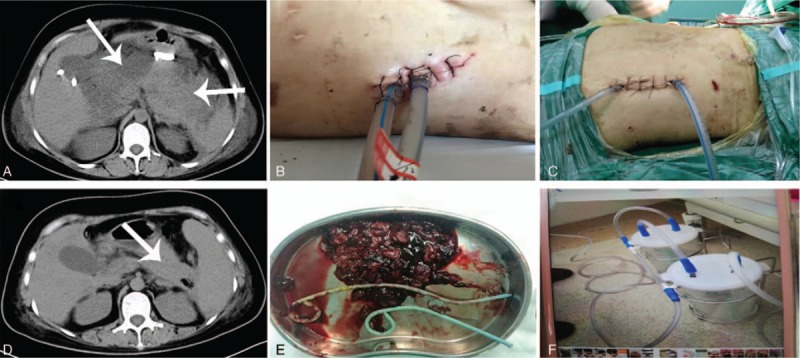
(A) Computed tomography before the first necrosectomy; (B and C) small incision minimally invasive approach; (D) computed tomography after last necrosectomy; (E) infected necrotic tissue; (F) negative pressure flushing device.
